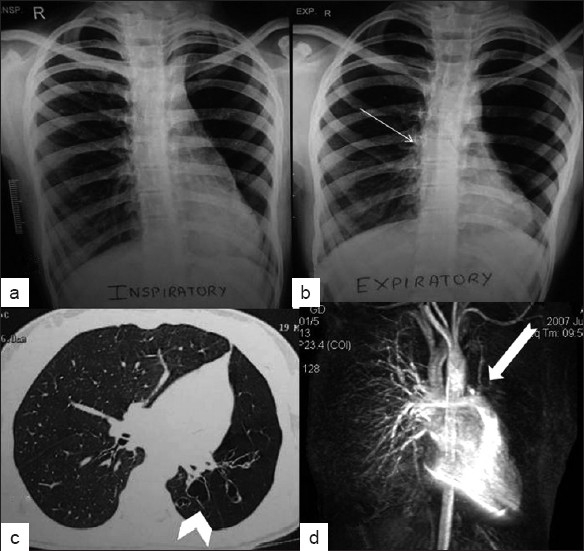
Inspiratory (a) and expiratory (b) chest radiograph of 13 year old girl showing small hyperluscent left hemithorax with mild mediastinal swing towards normal (right) side(thin white arrow). HRCT (c) demonstrates decrease in volume of left lung with decrease in bronchovascular markings on left side with bronchiectatic changes (white arrow head). MR angiography (d) confirming the diagnosis by revealing smaller pulmonary artery on left side with typical pruned tree appearance (thick white arrow)
